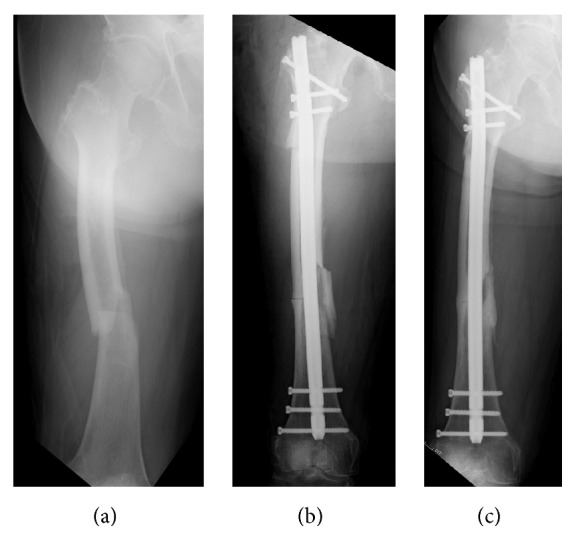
(a) AP radiographs of a patient with atypical femoral shaft fracture (b) treated with an intramedullary nail and intraoperative peri-implant fracture occurred. (c) Bone healed after 32 weeks from the index surgery.
